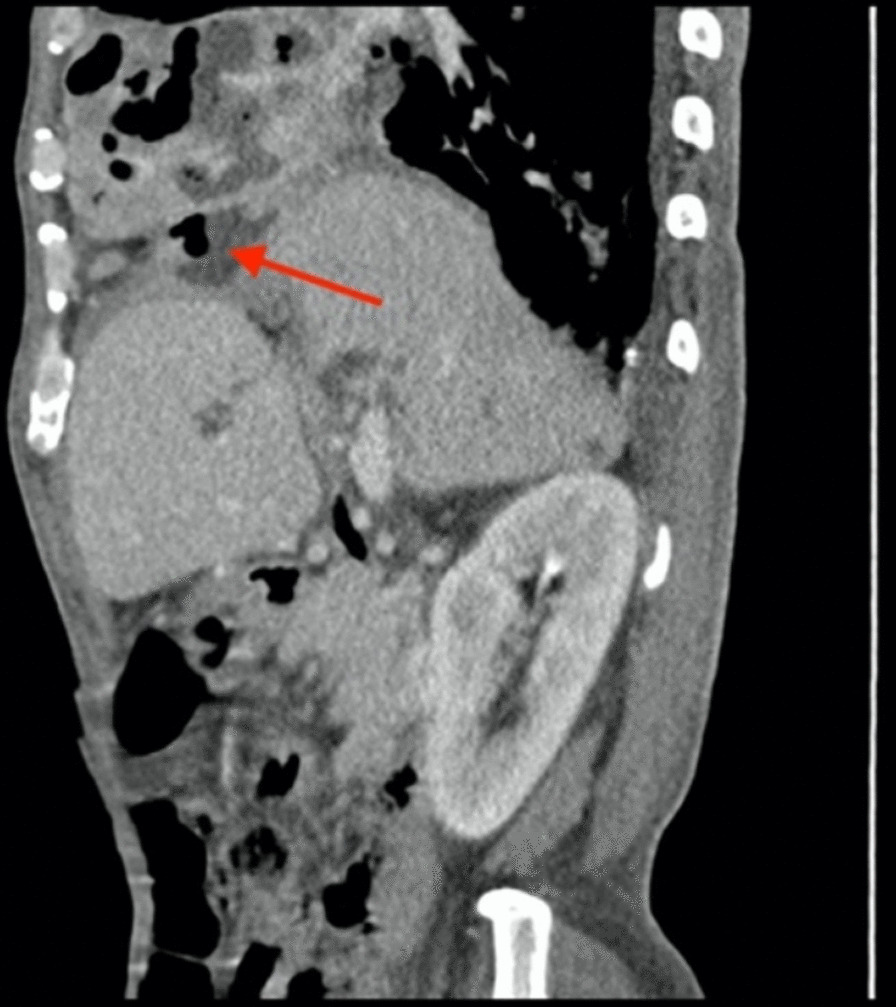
The BBF on the sagittal CT scan of the abdomen (red arrow)
